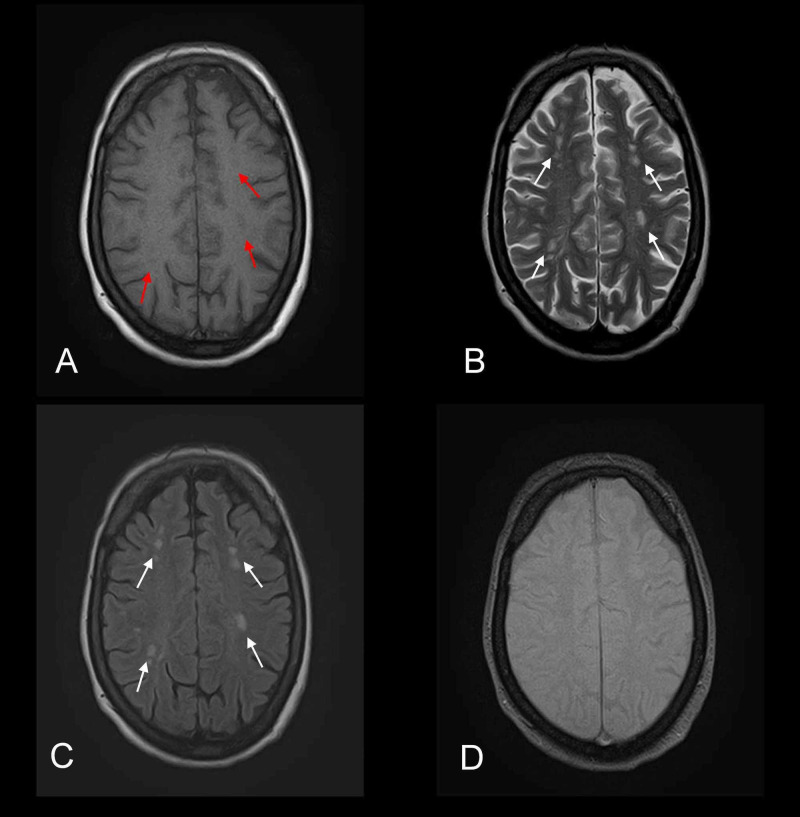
Axial MR images depicting microangiopathic ischemic changes consistent with small vessel pathology Multiple foci are noted distributed bilaterally in the frontoparietal regions and centrum semiovale. (A) Foci appear hypointense on axial T1-weighted MR image (red arrows). (B and C) Same foci appear hyperintense on axial T2-weighted and FLAIR MR images (white arrows). (D) GRE sequence does not show any bleed. MR, magnetic resonance; FLAIR, fluid-attenuated inversion recovery; GRE, gradient-echo.
